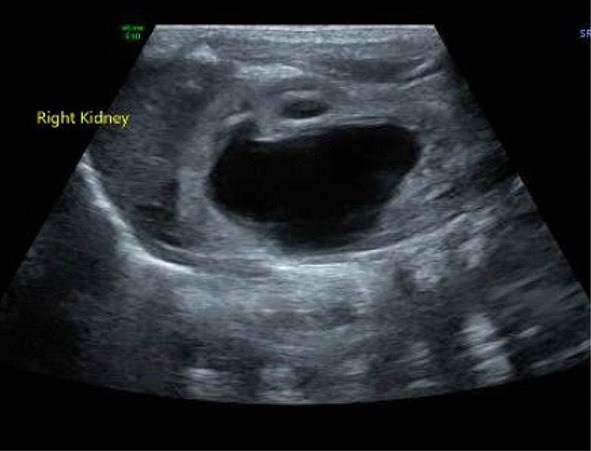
Ultrasound of the right kidney, demonstrating significant hydronephrosis with calyceal blunting and non-visualization of the right proximal ureter.
